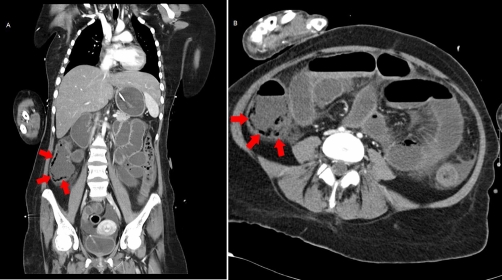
Abdominal and thoracic computer tomography of patient 1 coronal view: Multiple air filled cysts in the intestine wall along the right hemicolon can be seen (red arrows). Diagnosis Pneumatosis cystoides intestinalis was made. The sonographic findings (free abdominal air near the portal vein) were not confirmed in CT.
